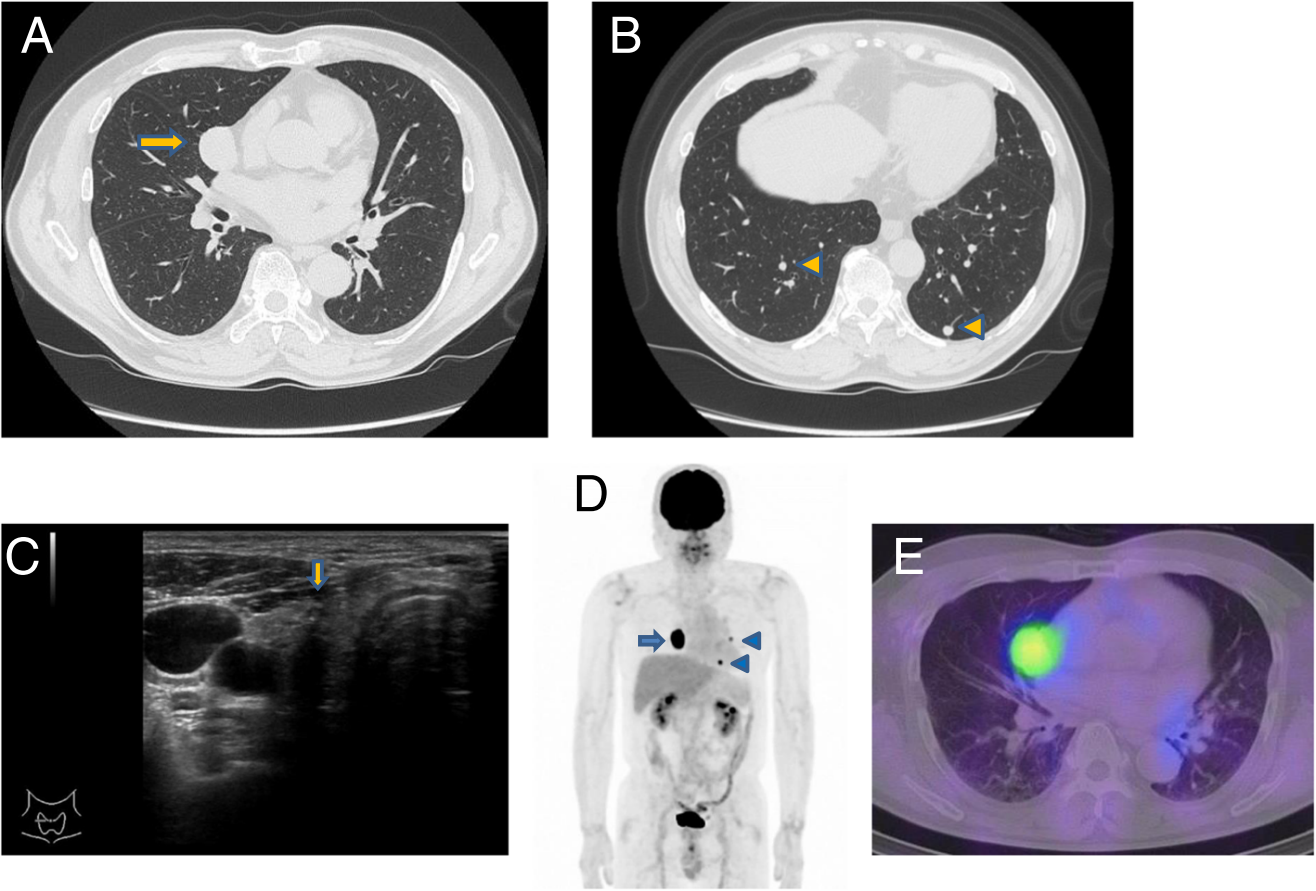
A, B: Computed tomography findings in the lung. A solid 30 mm mass (arrow) in the middle lobe of the right lung (A) and several 5 mm nodules (arrowheads) (B) can be seen in both lungs. C: Ultrasonographic findings in the thyroid gland. A hypoechoic mass measuring 8.6 × 4.6 mm and showing an indistinct border can be seen in the right lobe of the thyroid gland (arrow). D: ^18^F-fluorodeoxyglucose positron emission tomography (FDG-PET) findings. Strong FDG uptake is detected in the right lung tumor (arrow) and multiple tumors in the left lung (arrowhead). E: 131-iodine scintigraphy findings after the second radioactive iodine (RAI) treatment. 131-iodine has accumulated in the metastatic tumor in the middle lobe of the right lung
